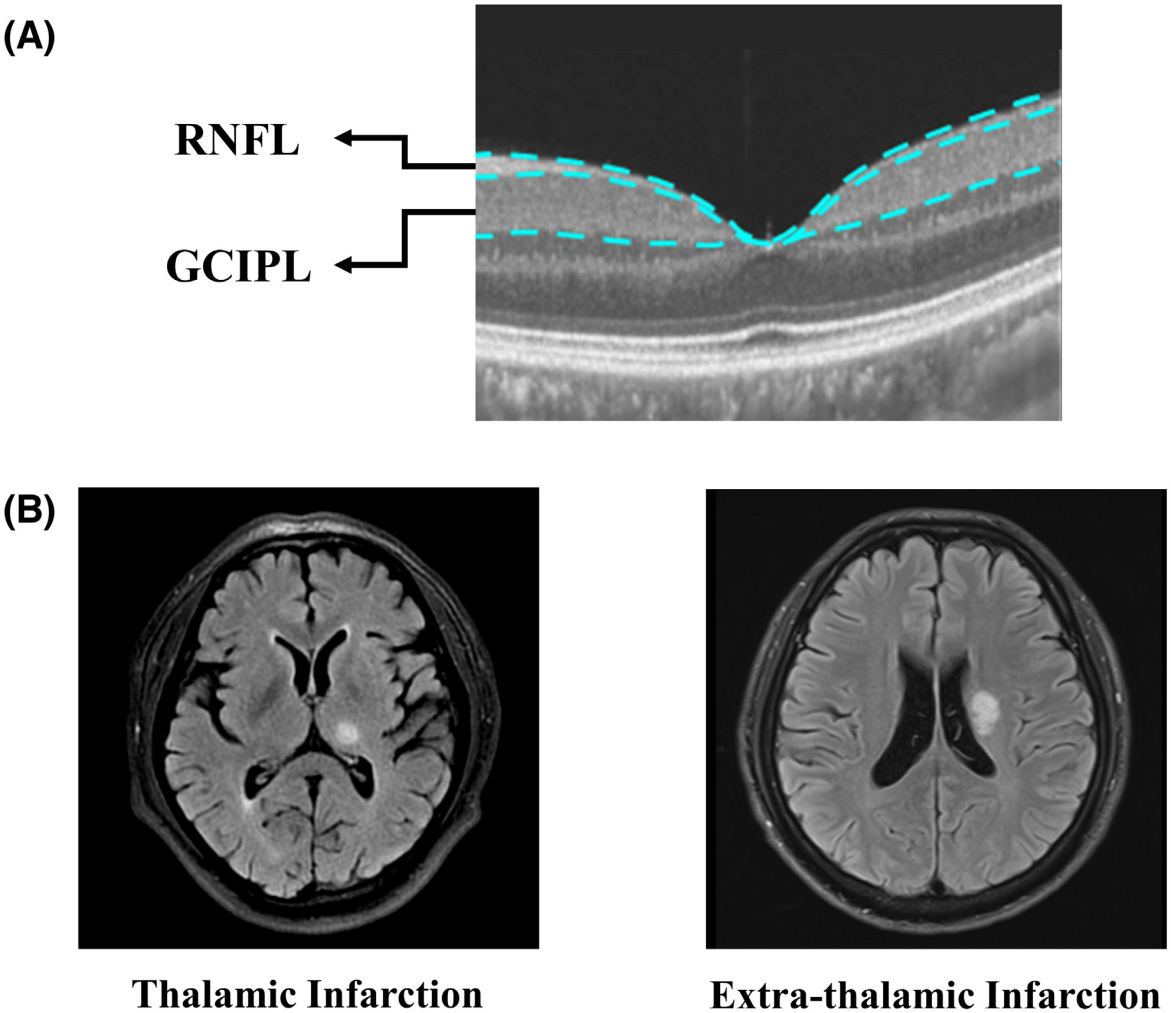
Representative images of OCT scans and MRI of patients. (A) The image shows the retina in a 3 × 3 mm^2^ area around the fovea. The OCT tool automatically segments the retina structure into RNFL and GCIPL. RNFL was defined as the thickness between the base of the inner limiting membrane (ILM) and the top border of the ganglion cell layer (GCL), and GCIPL was defined as the thickness from the base of the RNFL to the top border of the inner nuclear layer (INL). (B) The right image shows a fluid‐attenuated inversion recovery (FLAIR) MR image of a patient with thalamic infarction, and the left image shows an FLAIR image of a patient with extra‐thalamic infarction.
